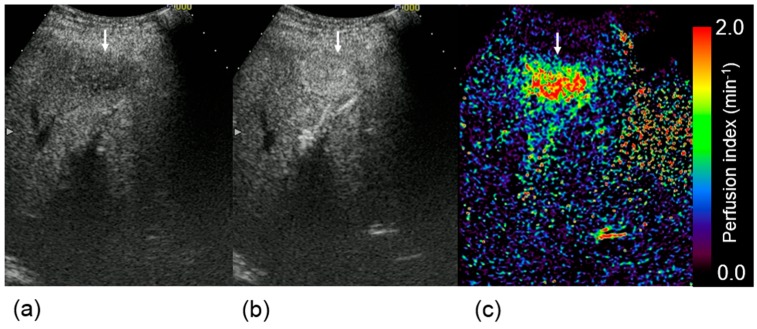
Dynamic contrast-enhanced ultrasound images obtained (a) before and (b) 30 s after contrast injection, and (c) a perfusion index map in the same subject. White arrows indicate hepatocellular carcinoma.
